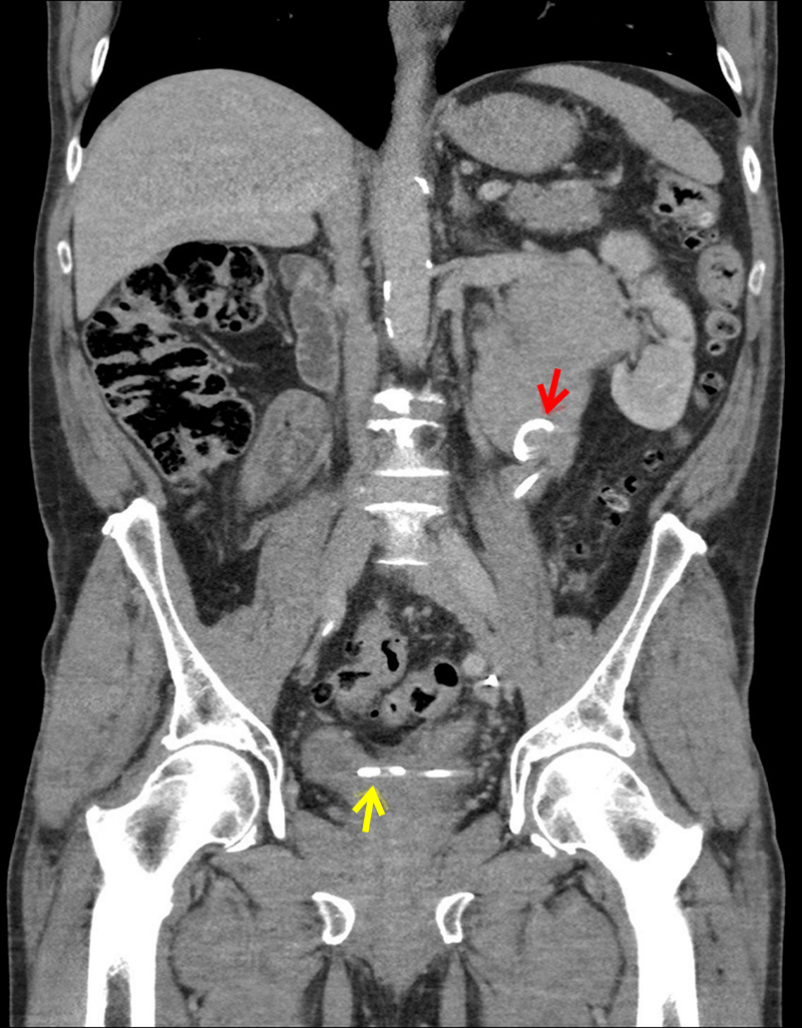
CT urogram showing stent (*red arrow* pointing to top end in dilated pelvis and *yellow arrow* to lower end in the bladder) in the baggy, distended system with no retroperitoneal collection.
